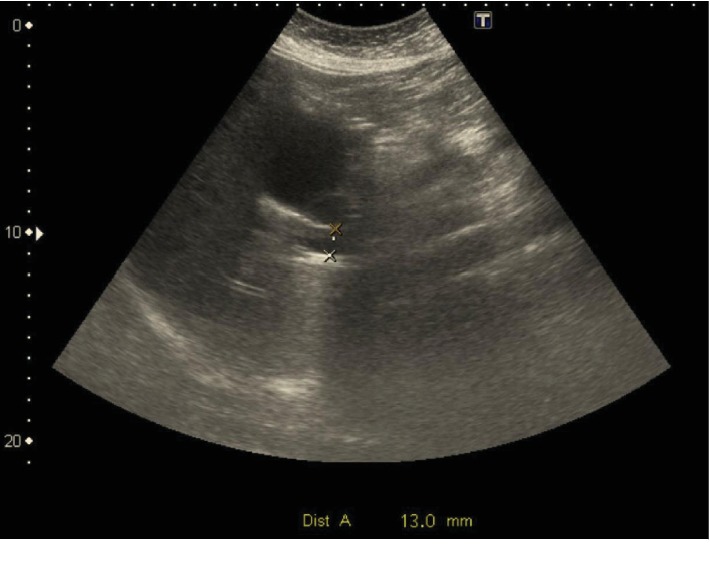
Transabdominal ultrasonography showing a dilated common bile duct (13 mm) with no visible choledocholithiasis.
